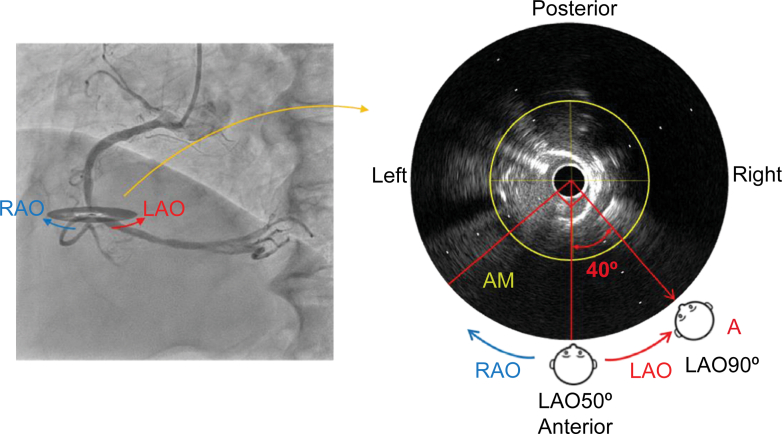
Determination of the Detector Position Enabling the Bifurcation to Be Most Clearly Distinguished On the angiographic image, right is LAO and left is RAO. On the IVUS image, counterclockwise is LAO and clockwise is RAO. The optimal angiographic view selected is LAO at 90°. AM = acute marginal; IVUS = intravascular ultrasound; LAO = left anterior oblique; RAO = right anterior oblique.
